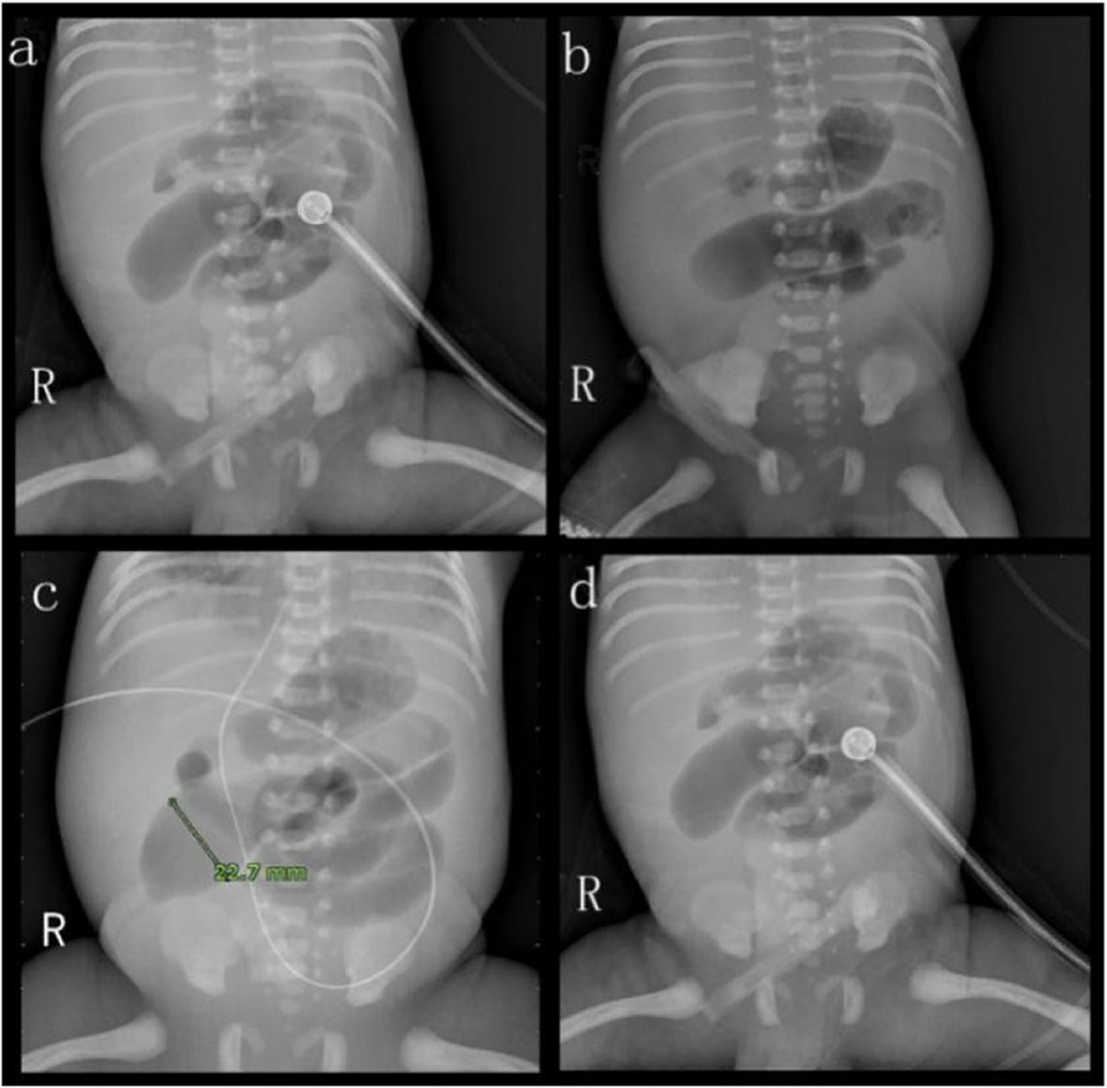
Multiple abdominal X-ray showed that the abdominal bowel was inflated very little and that the colon was not inflated (The examination times of a, b, c and d were consistent with the four chest X-rays)
